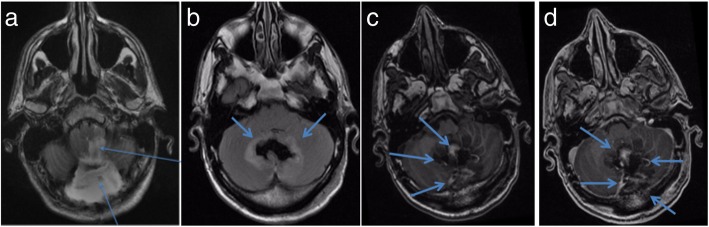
a axial T2-weighted, b axial FLAIR, c axial T1-weighted pre-contrast and d axial T2-weighted post contrast imaging demonstrate resection cavity with some blood degradation products and early post-operative enhancement; edema surrounding the resection cavity; contusion and edema left tonsil; and pseudomeningocele (blue arrows)
